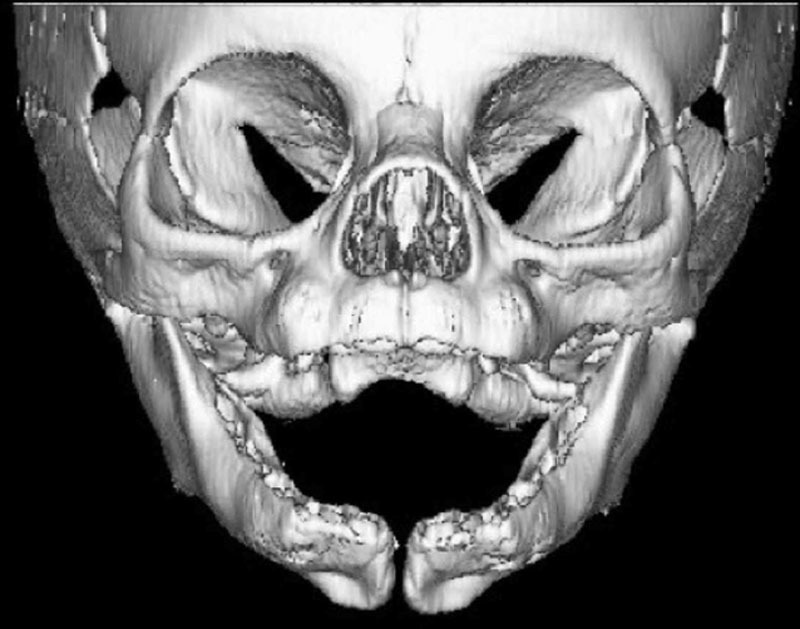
Preoperative computed tomography scan of face demonstrating median mandible defect.
